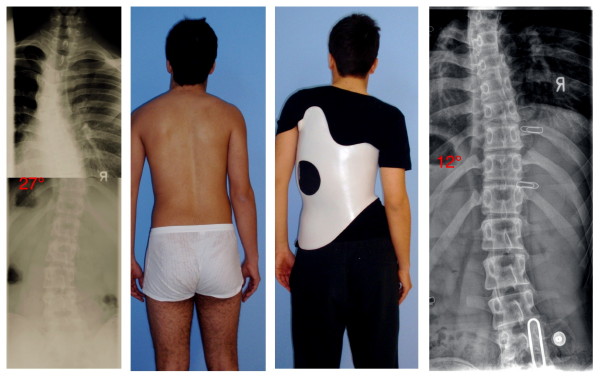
14-year old boy in his second Gensingen brace™ for the treatment of a double thoracic curvature. The initial angle of curvature at the start of treatment, when the boy was 12 years old, was 35° main thoracic with a significant high thoracic counter curve exceeding 30° as well. Now in his second Gensingen brace™, version 2010 for the treatment of a double thoracic curvature we have achieved a correction from 27° (intermediate result) to 12° in the main thoracic curvature, which is excellent considering the fact, that double thoracic curves cannot be corrected as easy as single thoracic curves. The foam pad location is marked on the in-brace x-ray with wire as is the longitudinal width of the thoracic pad.
